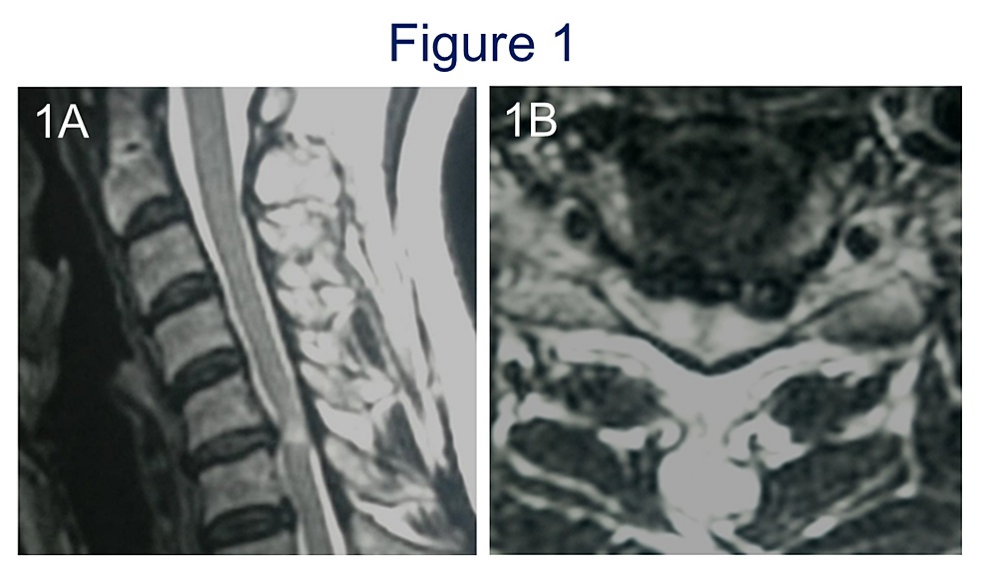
Preoperative T2 MRI sagittal view and axial view at C5-6 of case 1. MRI sagittal view showed canal stenosis at the C5-6 level (A) and axial view revealed a herniated C5-6 intervertebral disc (B).  High signal intensity was seen in the spinal cord at C5-6 disc level.
